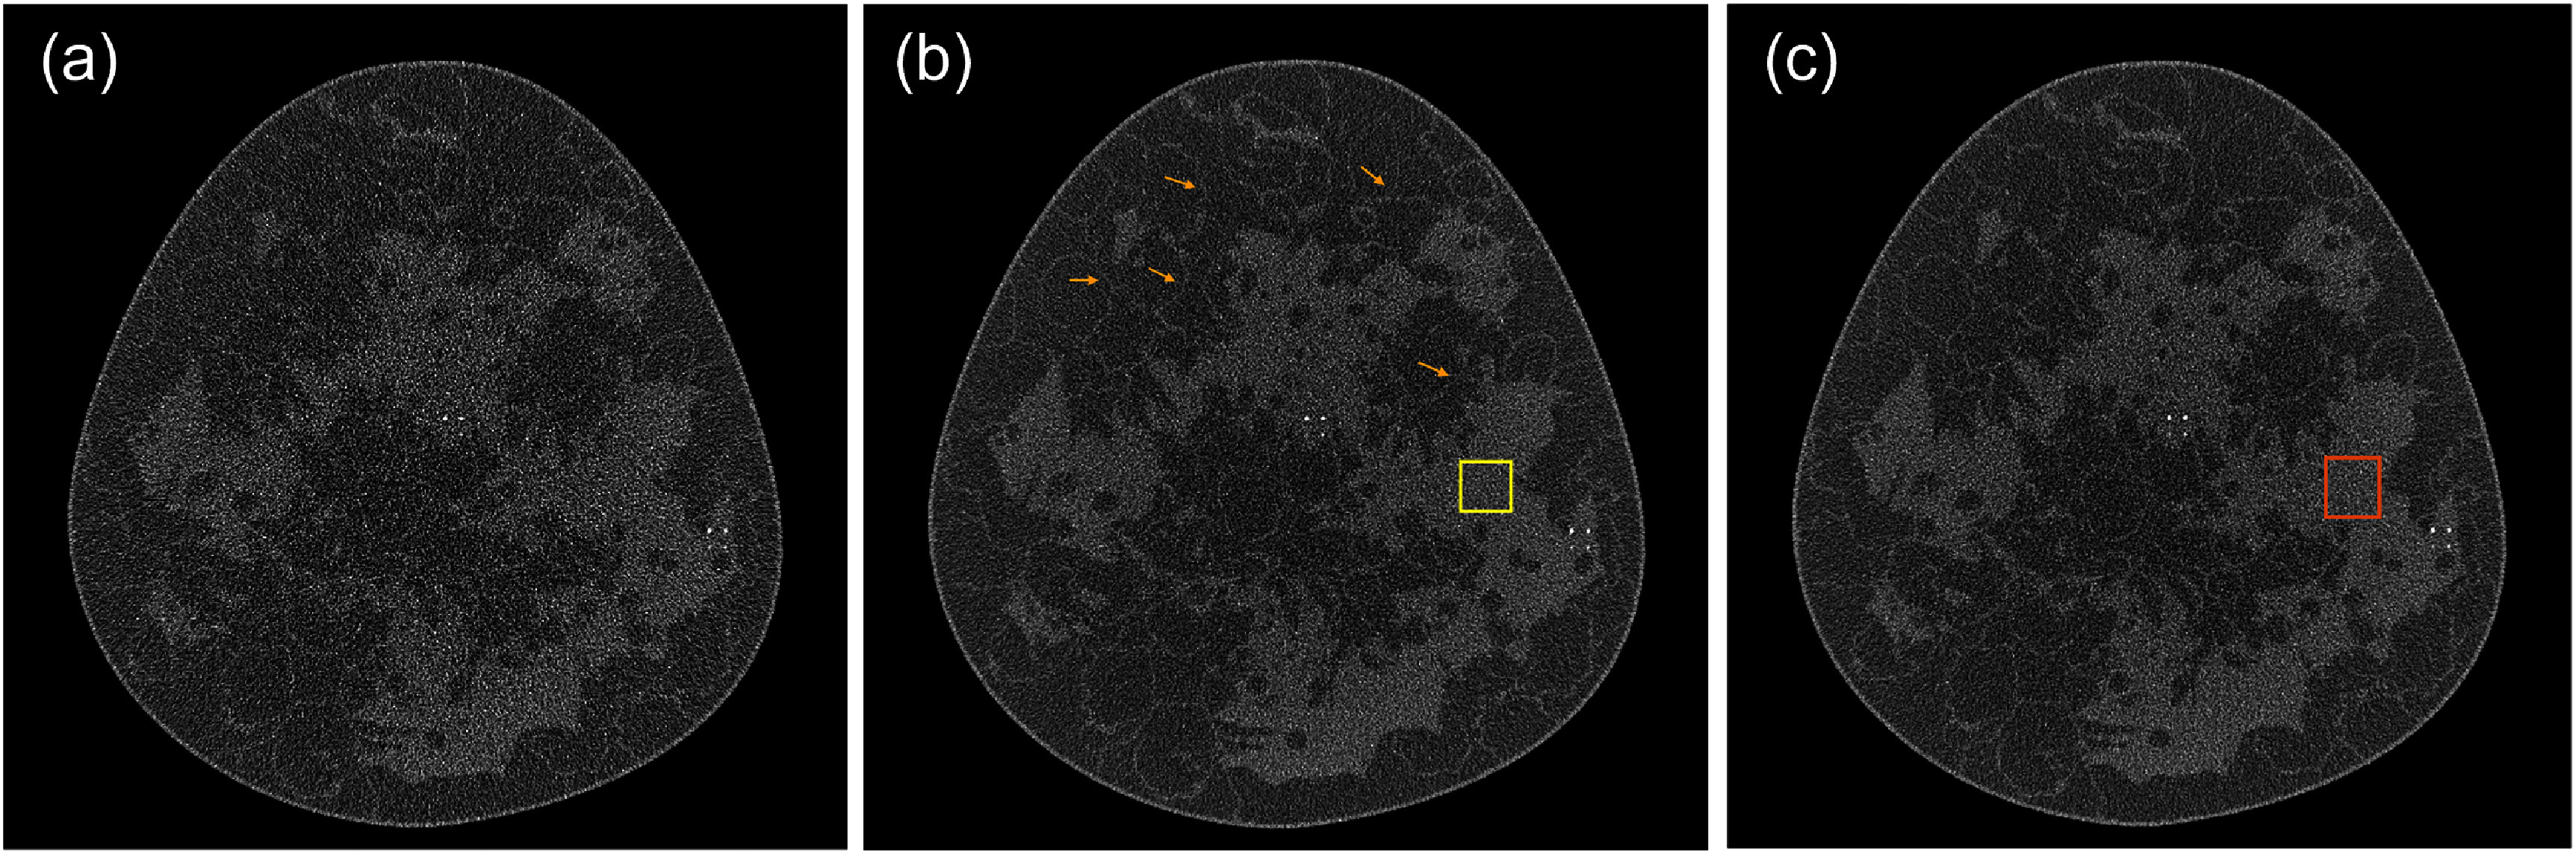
Representative reconstructed EID- and PCD-bCBCT images (display window: [0.1–0.4] cm^−1^). (a) EID-bCBCT image reconstructed using all photons. (b) PCD-bCBCT image reconstructed using all photons. Square is the ROI for calculating SD. Arrows indicate fine structures preserved in PCD-bCBCT image but lost in EID-bCBCT image. (c) PCD-bCBCT image reconstructed using primary photons only.
